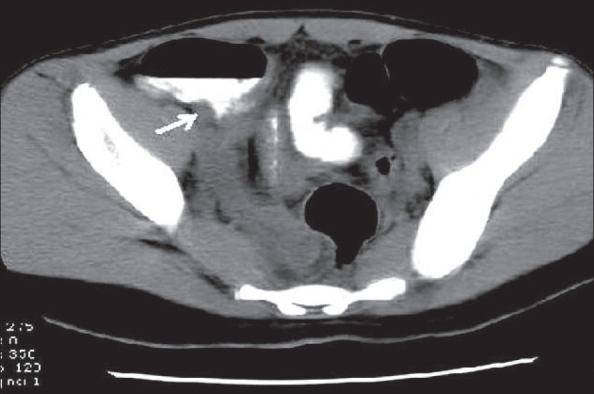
Arrowhead sign. Plain CT scan shows an arrowhead-shaped contrast collection in the cecum (arrow) due to extension of inflammation from the appendix to the cecum. The inflamed appendix is also seen
